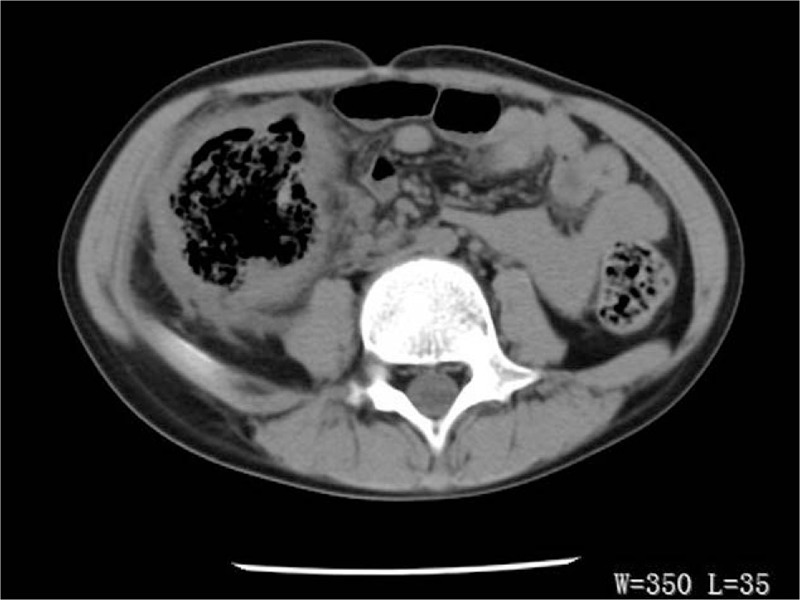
Computed tomography imaging demonstrated the bowel-wall thickening and luminal dilatation in the ascending colon.
